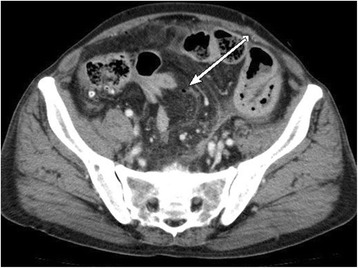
Computed tomographic findings: abdominal and pelvic computed tomography scan showing free air bubbles near the small intestine
